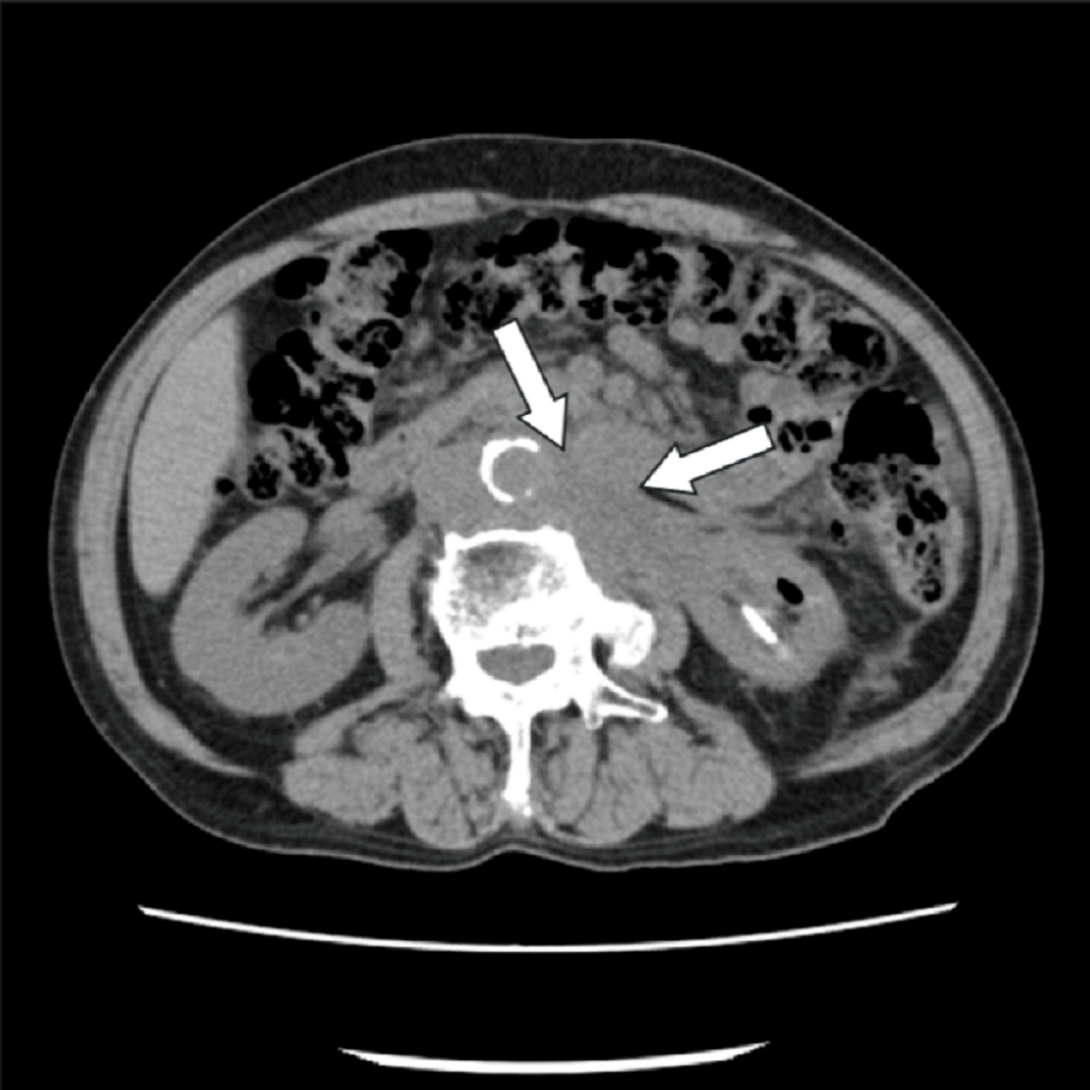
Metastatic sites in patient 4 Computed tomography imaging demonstrated para-aortic lymph node metastases, as indicated by arrows. A left percutaneous nephrostomy was subsequently performed.
